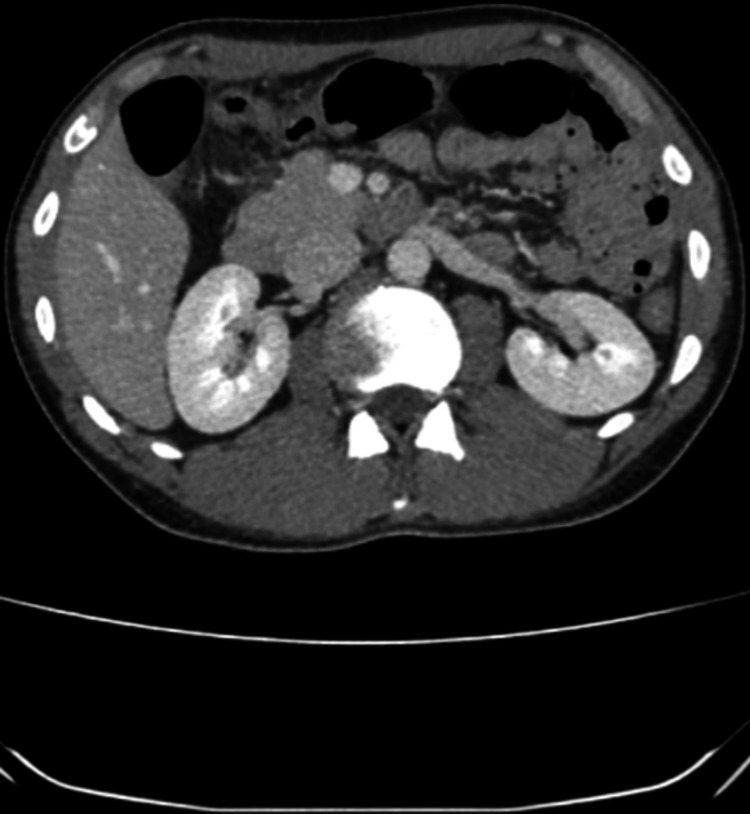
Stranding in the anterior abdominal wall in keeping with previous laparotomies. Normal appearances of the unprepared small and large bowels. No free fluid in the abdomen and pelvis. Normal appearances of the lung bases, adrenal, pancreas, liver, gall bladder, and kidneys. No cause was identified on CT imaging for the patient’s symptoms.
